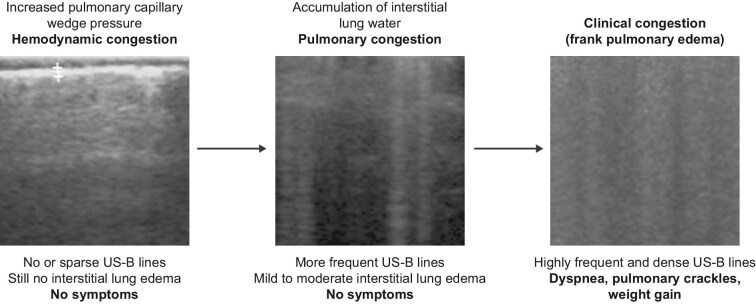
The imbalance of Starling's equilibrium at the alveolar-capillary level is central to generating congestion. Between haemodynamic and clinical pulmonary congestion, the intermediate event in the presymptomatic phase is an imaging sign detectable by lung ultrasound (US) as a US equivalent (US B-lines) to chest X-ray B-lines (Fig. 1). This sign may act as an indicator and an intermediate endpoint of frank pulmonary oedema.
